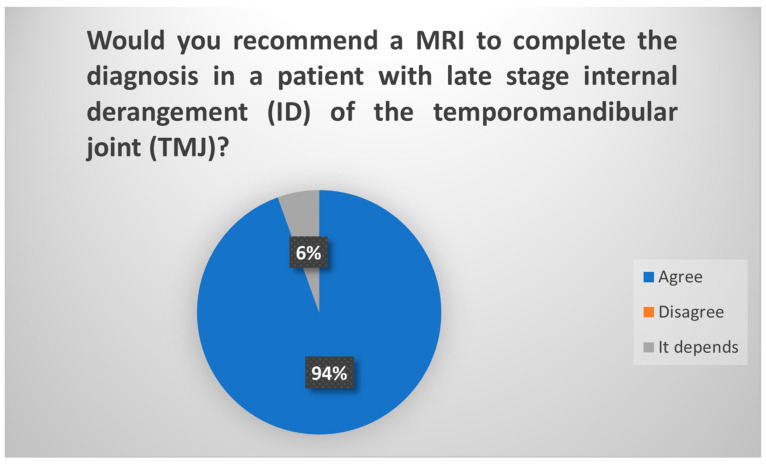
Delphi results regarding use of MRI in late-stage ID of TMJ. Consensus.
